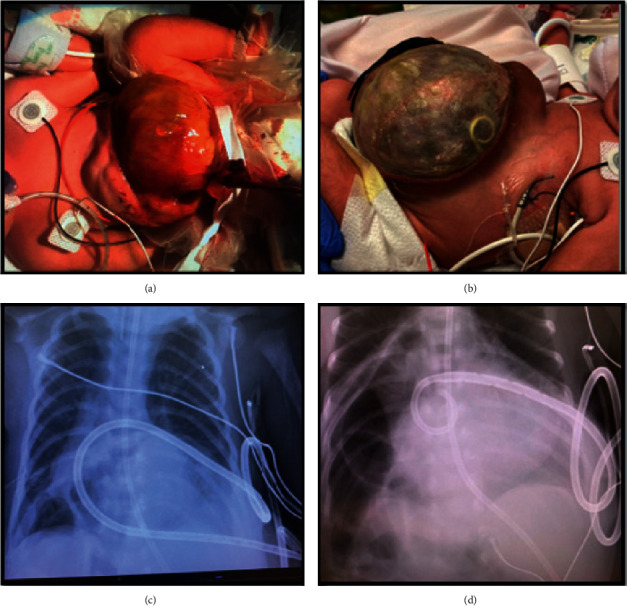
Omphalocele and drain images: (a) the patient's omphalocele at birth. (b) The Pigtail catheter placed in the pericardium is seen in the omphalocele sac, demonstrating continuity of the pericardium with the abdominal cavity. (c) Pericardial drain is seen in chest X-ray coursing around the cardiac silhouette and into the omphalocele. (d) The pigtail is retracted 2 cm with adequate draining and outside the omphalocele.
